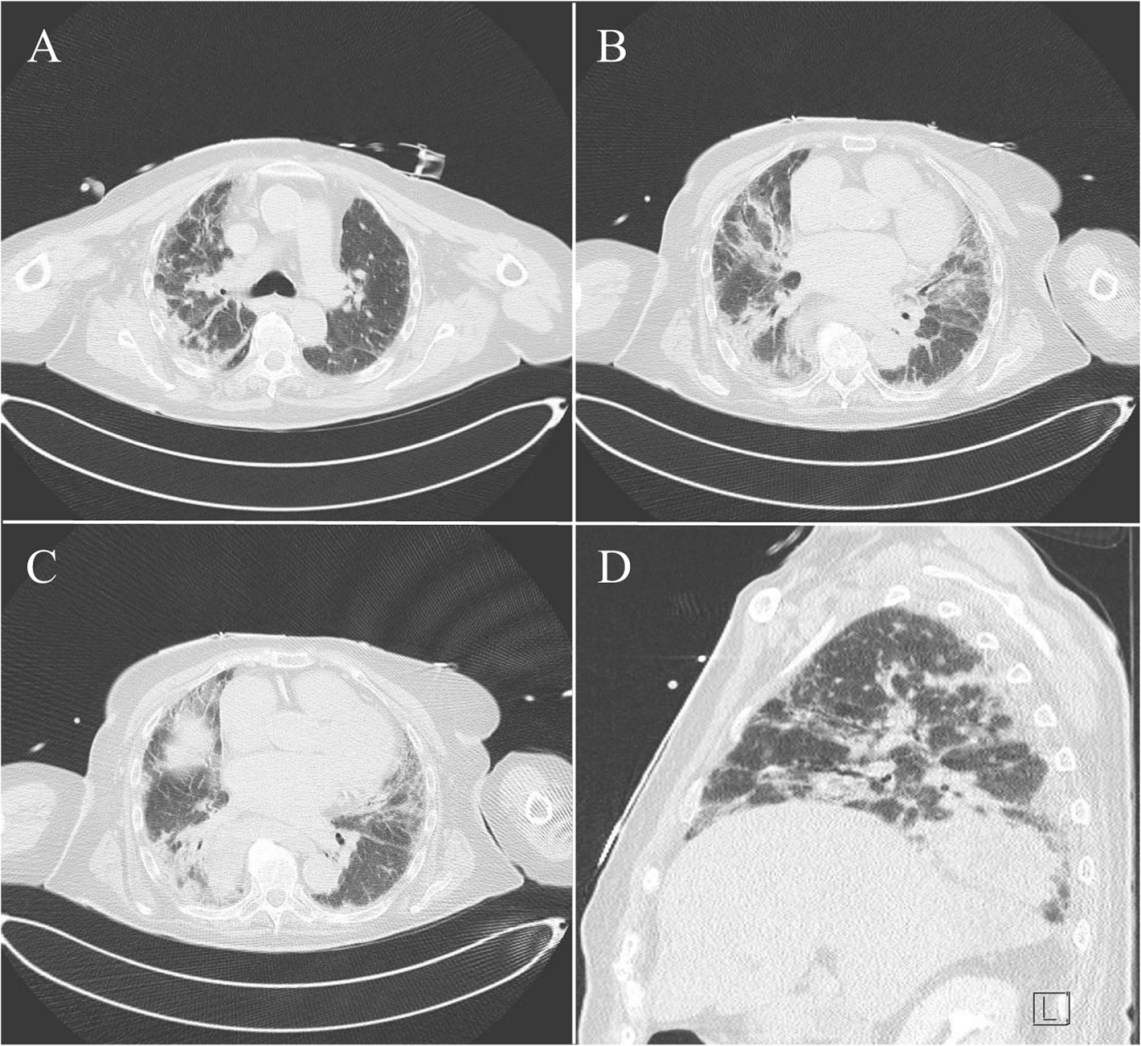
Patient’s chest CT showing ground glass opacities, predominantly peripheral, involving the five lung lobes, compatible with severe COVID-19 pneumonia. A–C Axial view. D Sagittal view
